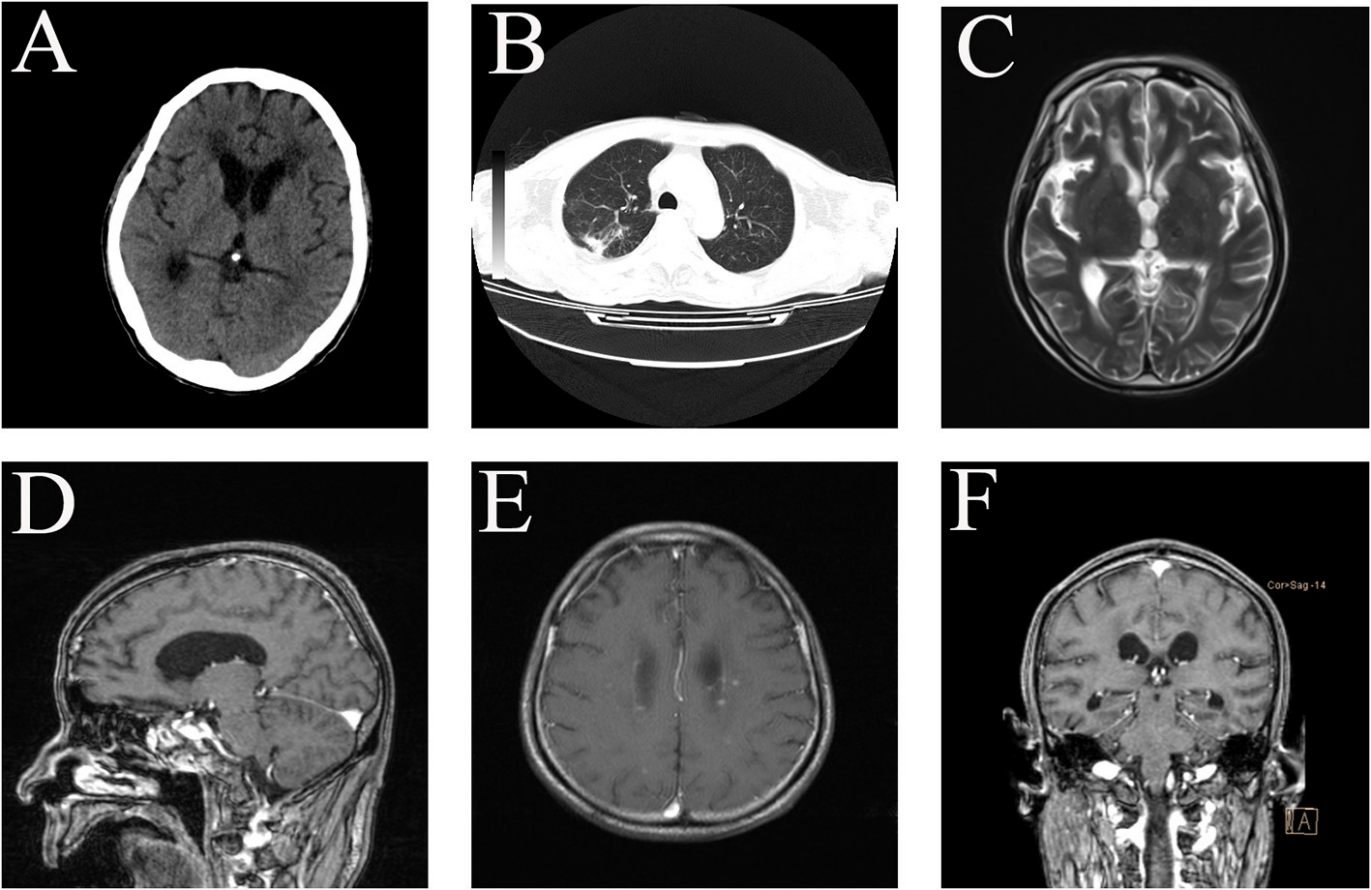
CT after 2 months of treatment. The size of the lesion and the degree of edema improved significantly (A). The patient's upper right pneumonia is better than before (B). The patient MRI in August 2021 showed that the abscess was significantly absorbed and improved (C–F).
